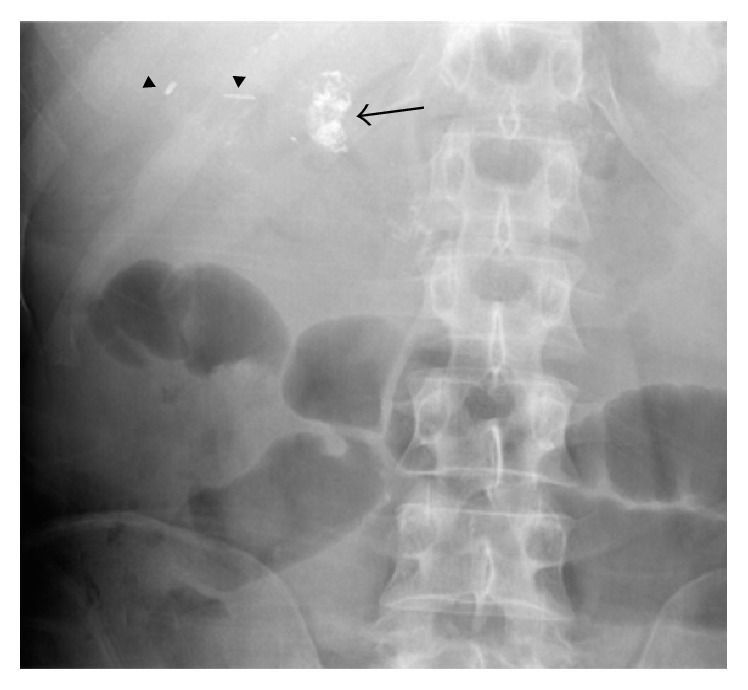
Plain radiograph of the abdomen following injection of the ectopic varix shows radiopaque embolic material (arrow) medial to surgical clips (arrowheads) positioned inferiorly to the right lobe of the liver.
